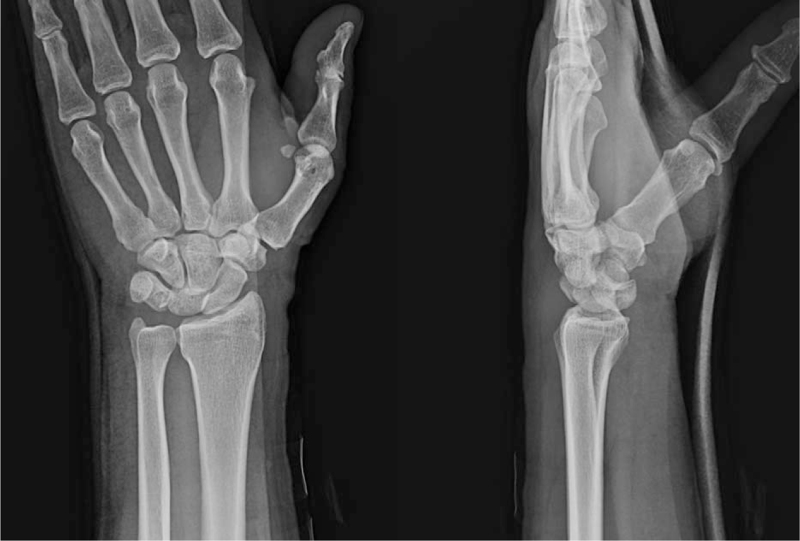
Posteroanterior and lateral wrist radiographs demonstrated a dorsal PLD. PLD = perilunate dislocation.
